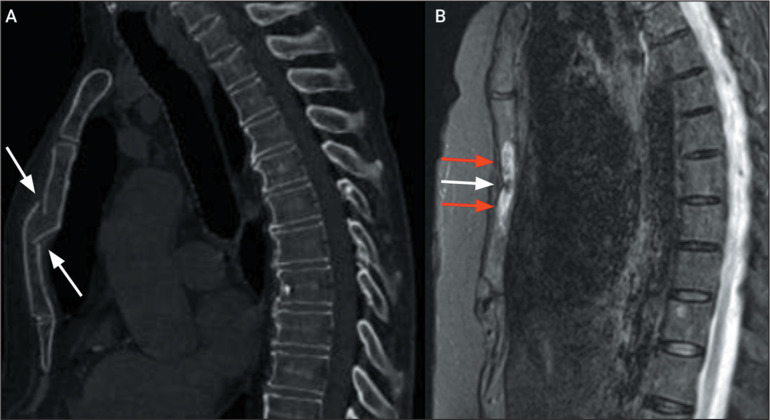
Acute sternal fracture. A: Sagittal CT scan of the chest, with bone
